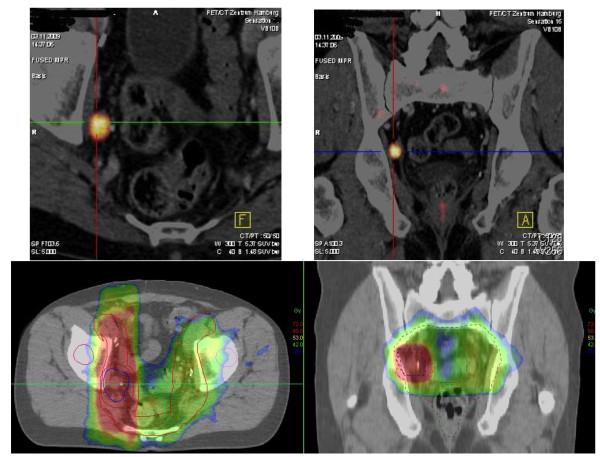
Dose distribution of FEC-PET/CT planned IMRT. An example of F18-fluoroethylcholine PET/CT-planned IMRT is shown (patient number 24, Table 1). PET/CT- fused images (upper part) showed a single positive lymph node in the right fossa obturatoria with an SUV _max _of 6,0 and an maximum diameter of 1,6 cm. The patient received IMRT irradiation of the pelvic lymph nodes to 45 Gy (five weekly fractions of 1,8 Gy) with a total boost dose of 66,6 Gy applied to the lymph node metastases (lower part of figure; colour wash images of transversal and coronal plane are depicted. Green: 42 Gy; red to orange: 60 to 72 Gy).
